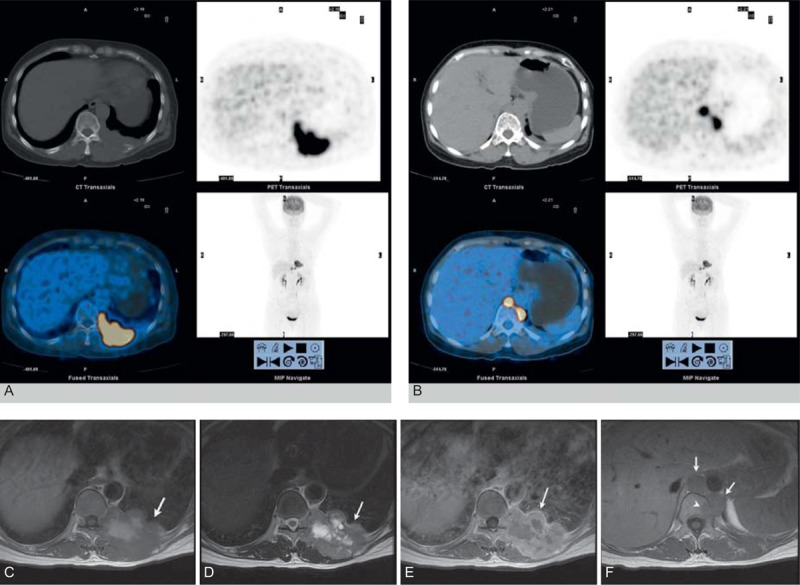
A 20-year-old man presented with a history of left back pain for about 1 month and fever for 5 days. FDG-PET/CT images (A, B) show increased metabolic activity in the left chest wall mass, the retrocrural lymph nodes, and bony destruction. The current SUVmax of this lesion was 18.6. The T1-weighted image (C) and the T2-weighted image (D) show that the tumor had heterogeneous intermediate and high signal intensity (arrow). The enhanced T1-weighted image (E) shows that the tumor had intermediate heterogeneous enhancement (arrow). In addition, at the 4-month follow-up after surgery and chemotherapy, the T1-weighted image (F) shows that retrocrural lymph nodes were enlarged (arrow) and the bodies of thoracic vertebrae were destroyed (arrowhead).
